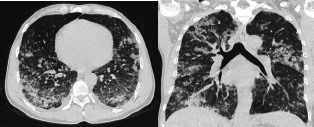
HRCT chest shows multiple areas of ground-glass opacities with a crazy-paving pattern, centrilobular nodules in branching pattern giving the appearance of tree in bud appearance.
